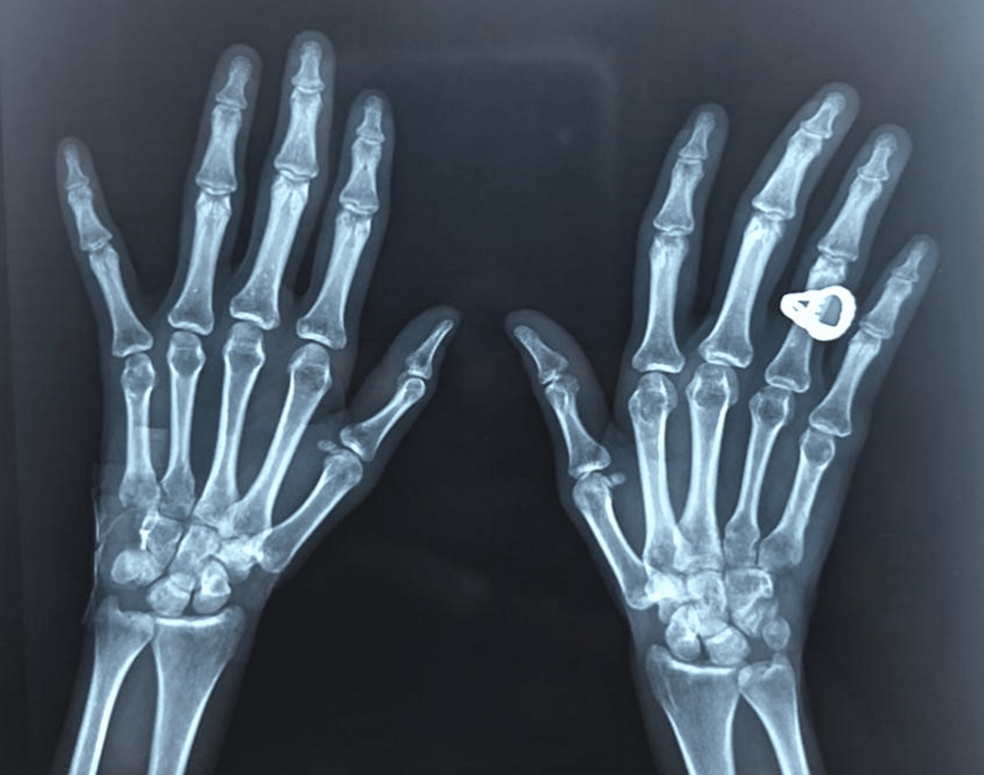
Hand X-ray X-ray showing normal symmetrical joints without bone overlapping except at the carpal and base of the metacarpal bones. No fractures, osteopenia, or bone deformities were noted.
